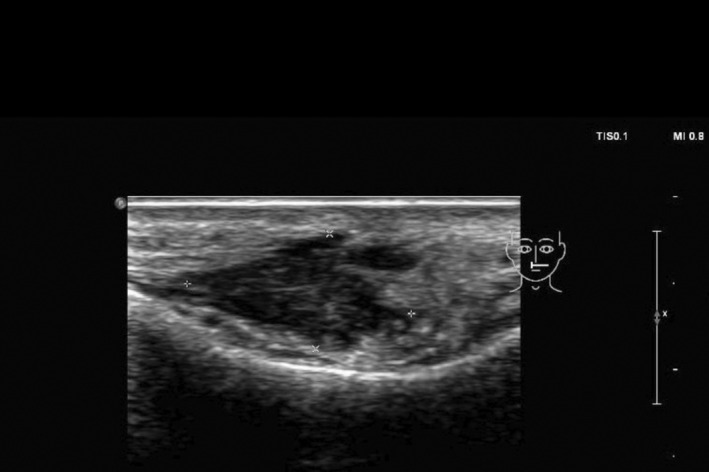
Ultrasonographic findings in patient 2 demonstrate ill‐defined, hypoechoic filler deposits within the deep subcutaneous fat in multiple locations, sometimes reaching the skeletal muscles.
